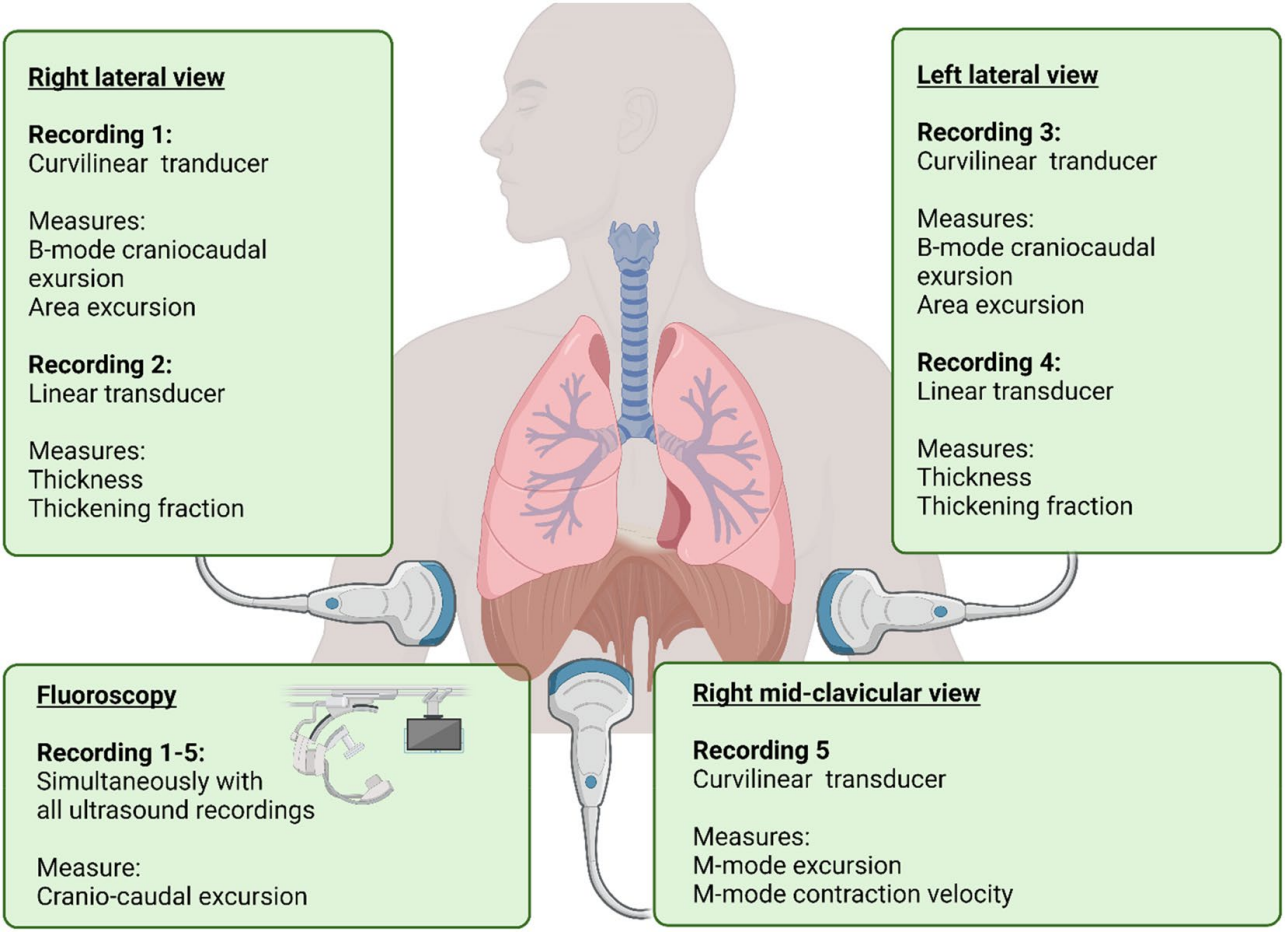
Study setup. With participants in sitting position, ultrasound and fluoroscopic recordings were made simultaneously. Ultrasound recorded diaphragm excursion from the left lateral view (recording 1 and 2 with curvilinear and linear transducer), from the right lateral view (recording 3 and 4 with curvilinear and linear transducer), and from the midclavicular view (recording 5 with curvilinear transducer). All recordings were made in both sniff and IC inspiration, a total of ten recordings. Fluoroscopy recorded diaphragm excursion in the same breathing manoeuvre as was recorded by ultrasonography. The recordings were later analyzed and the diaphragm excursion was measured as described in the figure and in the text
